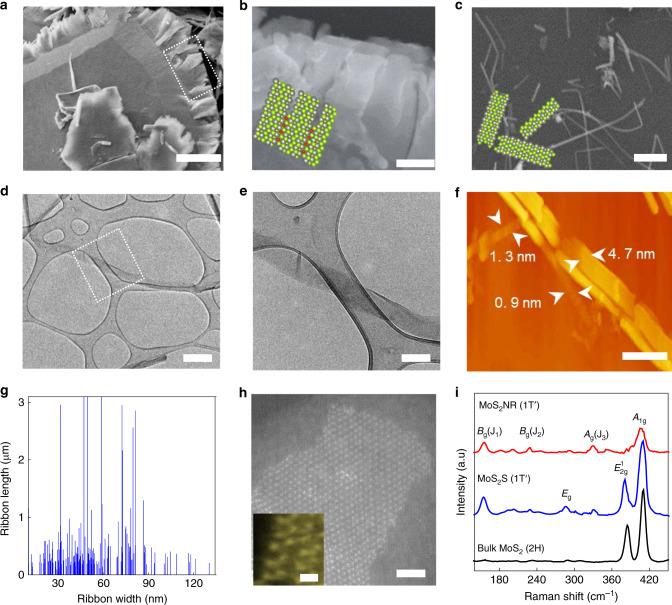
Unzipping of 2D MX_2_. SEM image of (a) unzipped MoS_2_ after 5 min of ultrasonication displaying the initiation of unzipping from flake edge, (b) Magnified image for unzipped edge and (c) Fully unzipped MoS_2_ displaying discrete MoS_2_ NRs. Scale bars, (a, c) 0.5 and (b) 0.1 µm. d, e TEM image of isolated MoS_2_ NRs. Scale bars, (d) 0.2 and (e) 0.1 µm (f) AFM image of MoS_2_ NRs. Scale bar, 0.2 µm (g) Plot of NR length as a function of width measured by TEM of more than 400 MoS_2_ NRs. h STEM image of MoS_2_ NR. Inset is the lattice image with low beam exposure displaying 2a0xa0 symmetry and Mo terminated zigzag edge structure. Scale bars, 1.5 and 0.15 nm (inset). i Raman spectra of bulk MoS_2_, 1T’ MoS_2_ sheets (MoS_2_ S) and MoS_2_ NRs.
